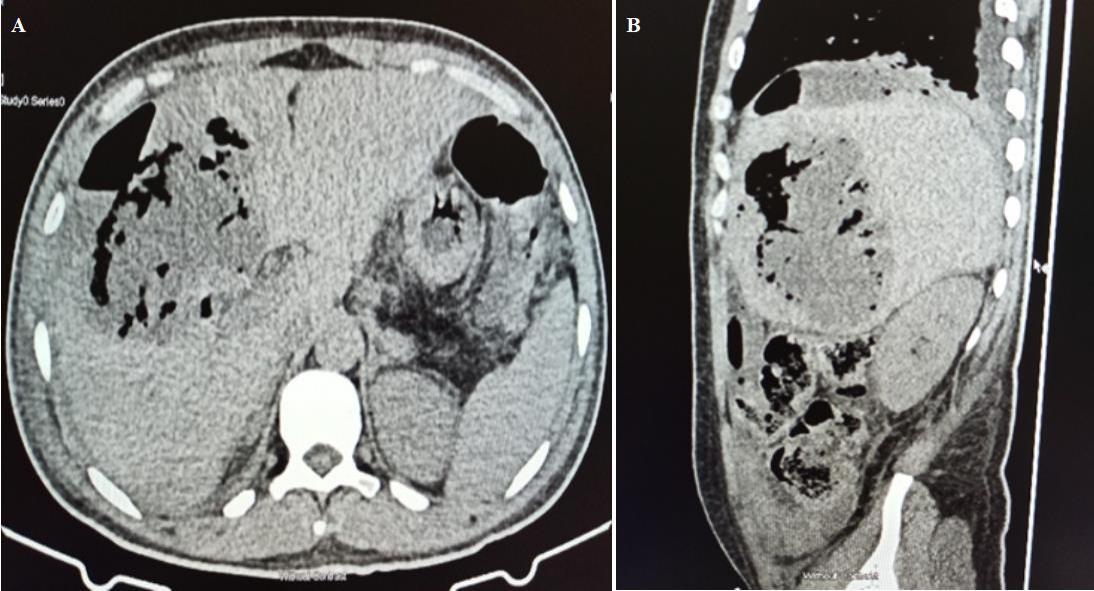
A,B) axial and sagittal non-contrast computed tomography scan of the abdomen showing a large gas-forming liver abscess in segments VII and VIII with peri-hepatic and intrahepatic air-fluid levels
